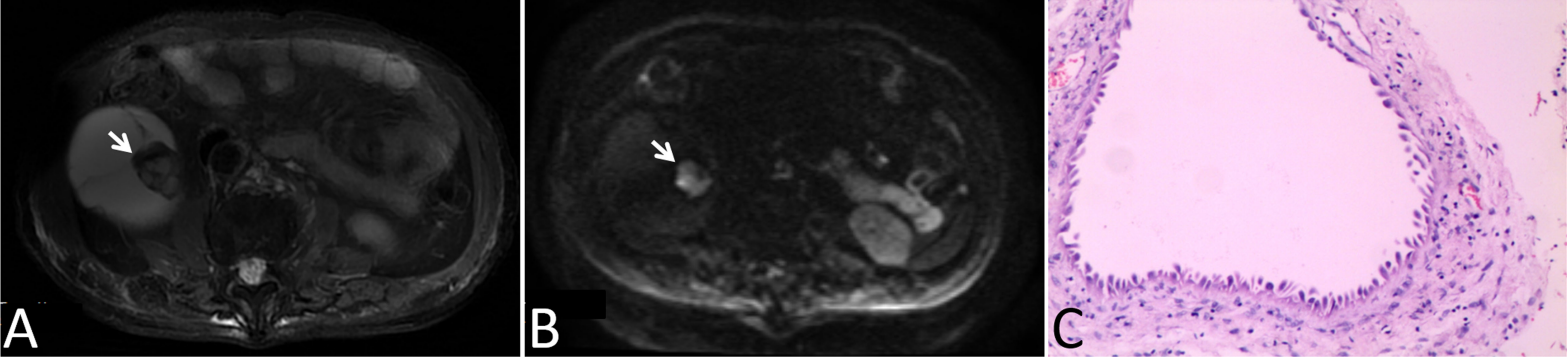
Images in a 78-year-old man with a cystic mass in the right kidney. (A) Axial T2-weighted MR image shown mural nodular soft tissue (arrow), (B) Axial DWI (b = 1000 s/mm2) image shown remarkably high signal intensity of the wall nodule (arrow), (C) This lesion was surgically removed and determined to be a multilocular cystic renal neoplasm of low malignant potential.
